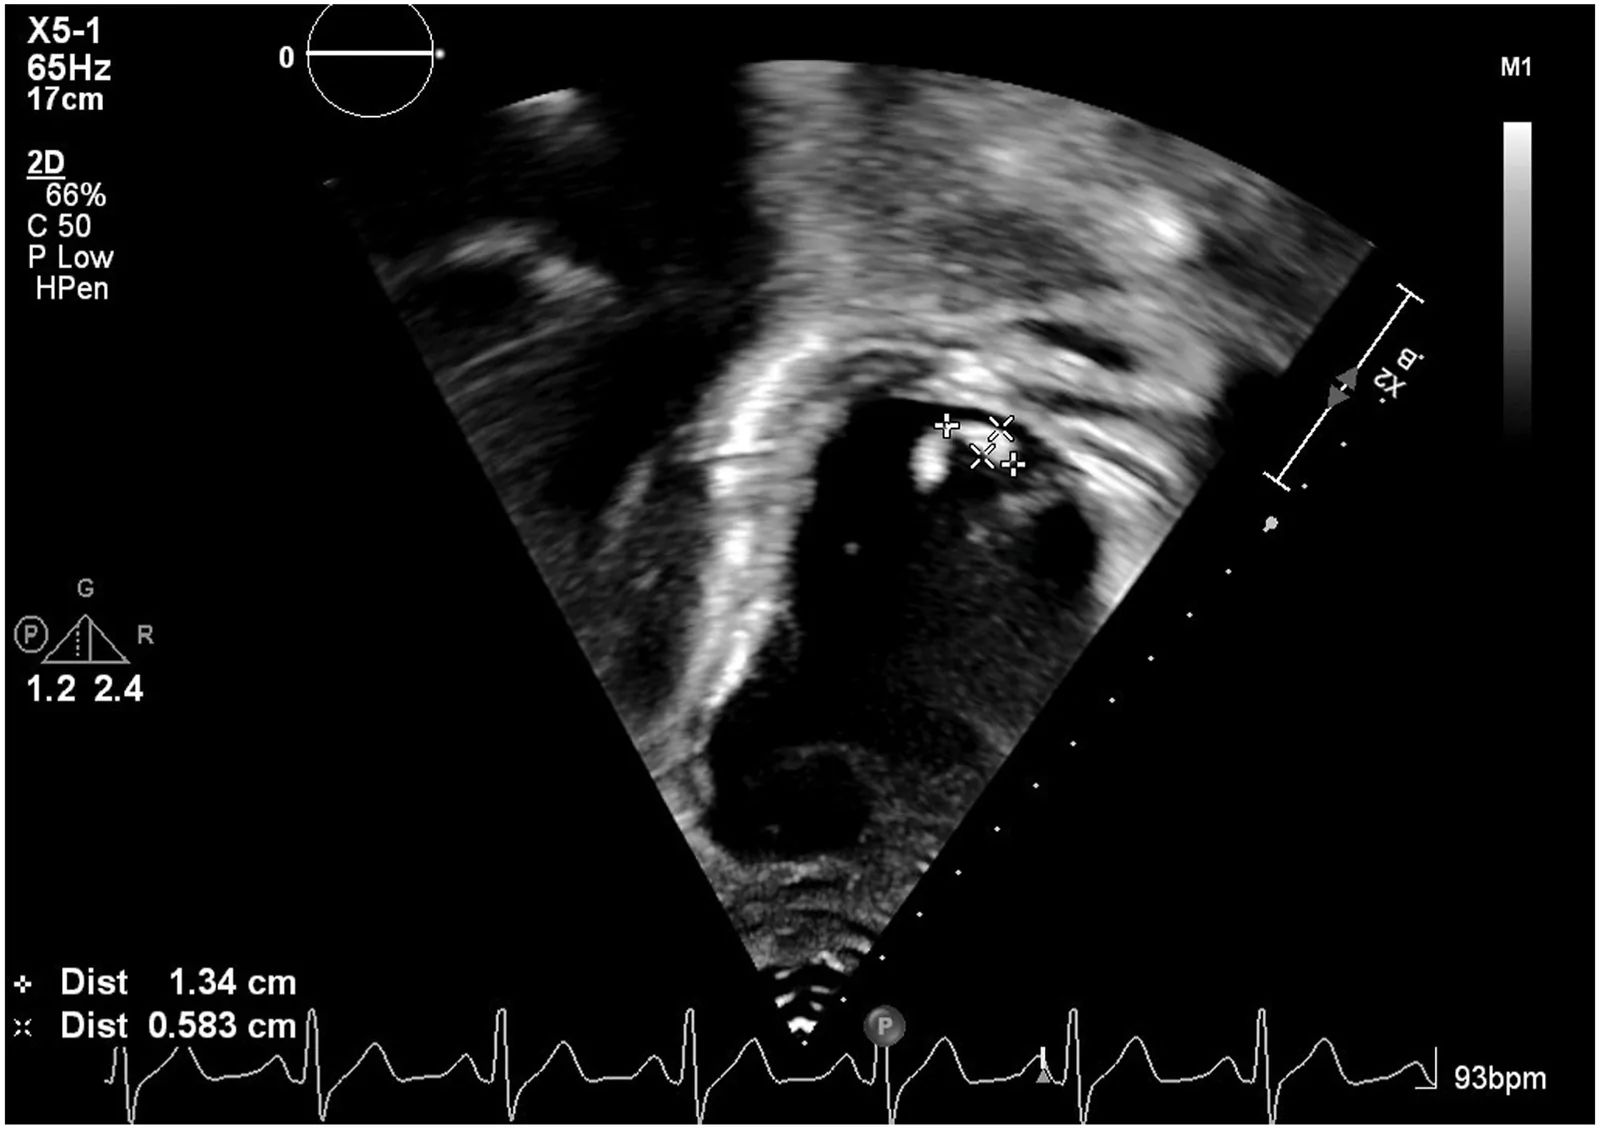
Two-dimensional TTE, apical two-chamber mid-diastolic view, demonstrates an irregular, echogenic mass (13.4 × 5.8 mm) on the atrial surface of the MV, consistent with a vegetation.
